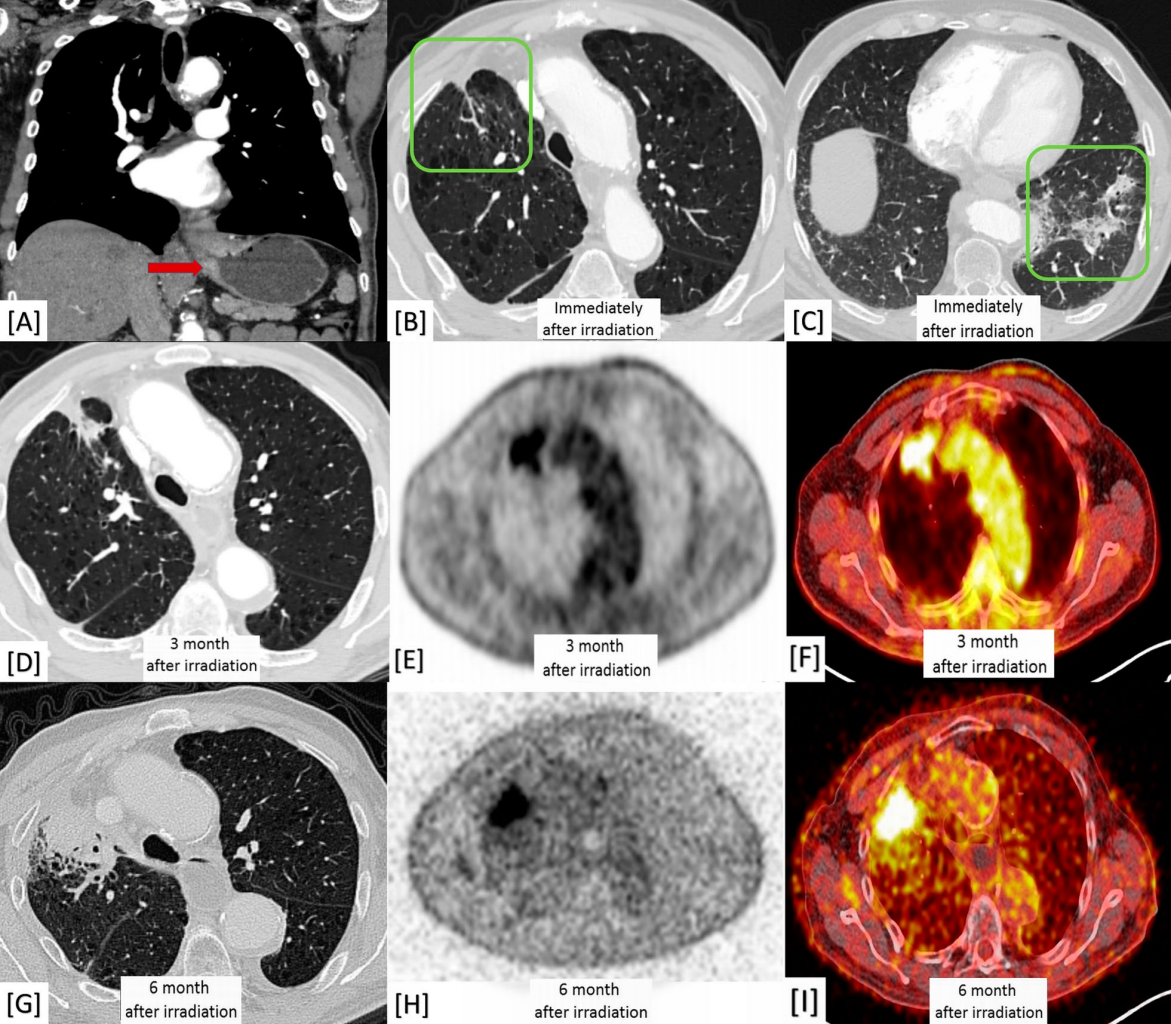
A 63-year-old male patient with a history of gastro-esophageal carcinoma managed by CRT. A Pre-management coronal chest CT (mediastinal window) expressing the gastro-esophageal malignant mural thickening (red arrow). B Axial chest CT (lung window) immediately after radiotherapy cycles showing lung emphysematous changes with right anterior upper lobar small scar showing speculated outlines (green square). C Axial chest CT (lung window) immediately after radiotherapy cycles showing left basal parenchymal scarring with ground-glass halo (green square). D Three-month post-irradiation axial chest CT (lung window) showing progression in size and peripheral speculations of the scar. E, F Three-month post-irradiation PET/CT scans showing high FDG-uptake. G Six-month post-irradiation axial chest CT (lung window) showing further progression in size and peripheral speculations of the scar with fleshy soft tissue appearance with interrupted air bronchogram. H, I Six-month post-irradiation PET/CT scans confirming size progression and showing higher FDG-uptake and higher SUV. Diagnosis: Proved patient with metastatic deposit
